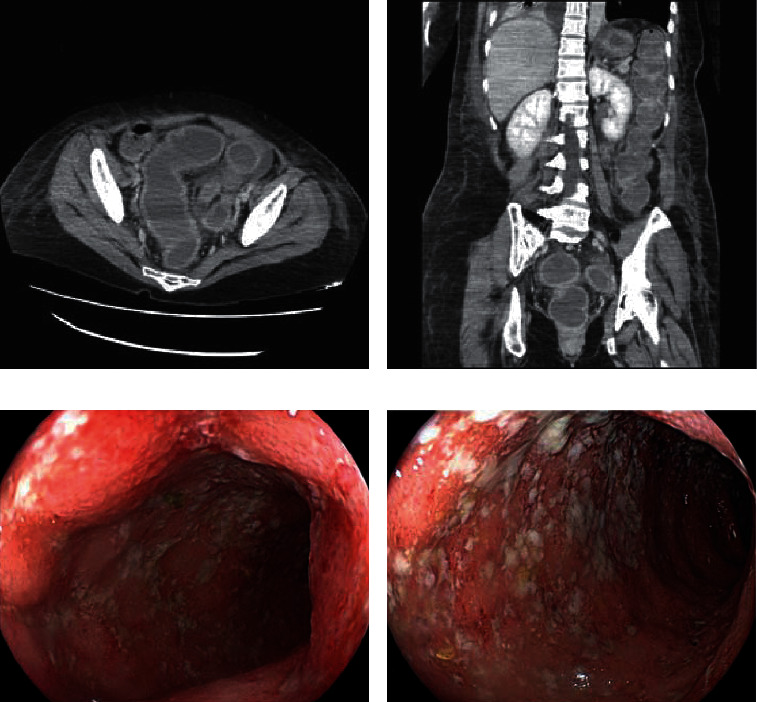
(a) Axial and (b) coronal images of contrast enhanced computed tomography (CT) showing colonic mural thickening and enhancement of the left-sided colon, consistent with colitis. Hyperattenuating material within the bowel lumen can be seen, suggestive of blood clots. Endoscopic findings of the (c) rectum and (d) sigmoid colon showing edematous mucosa with marked erythema, absent vascular pattern, friability, and erosions, which are consistent with ulcerative colitis (UC).
